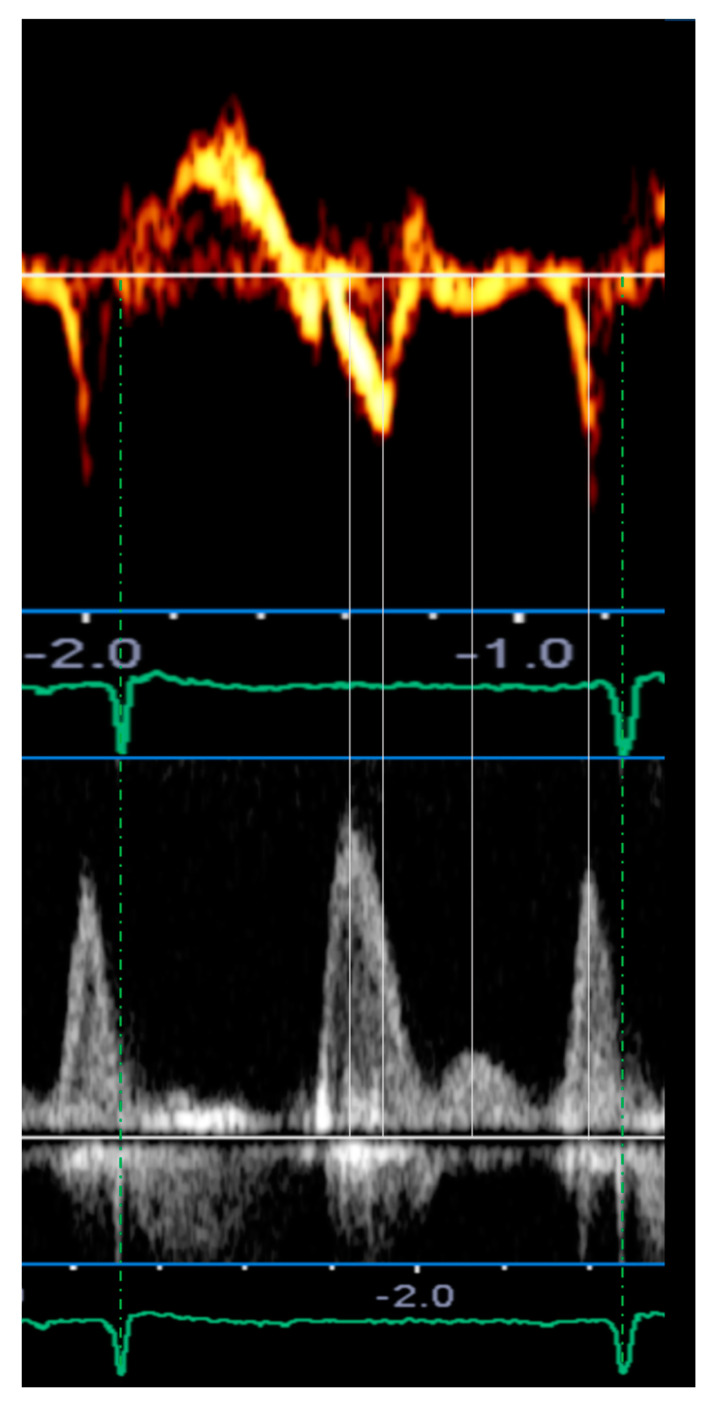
The upper and lower panels show septal mitral annular velocity by tissue Doppler imaging (TDI) and transmitral flow velocity in the pulsed-wave Doppler mode, respectively. The two Doppler spectra relate to two consecutive RR cycles which had the same duration of 1100 ms (dashed green lines), therefore a temporal comparison was possible.
